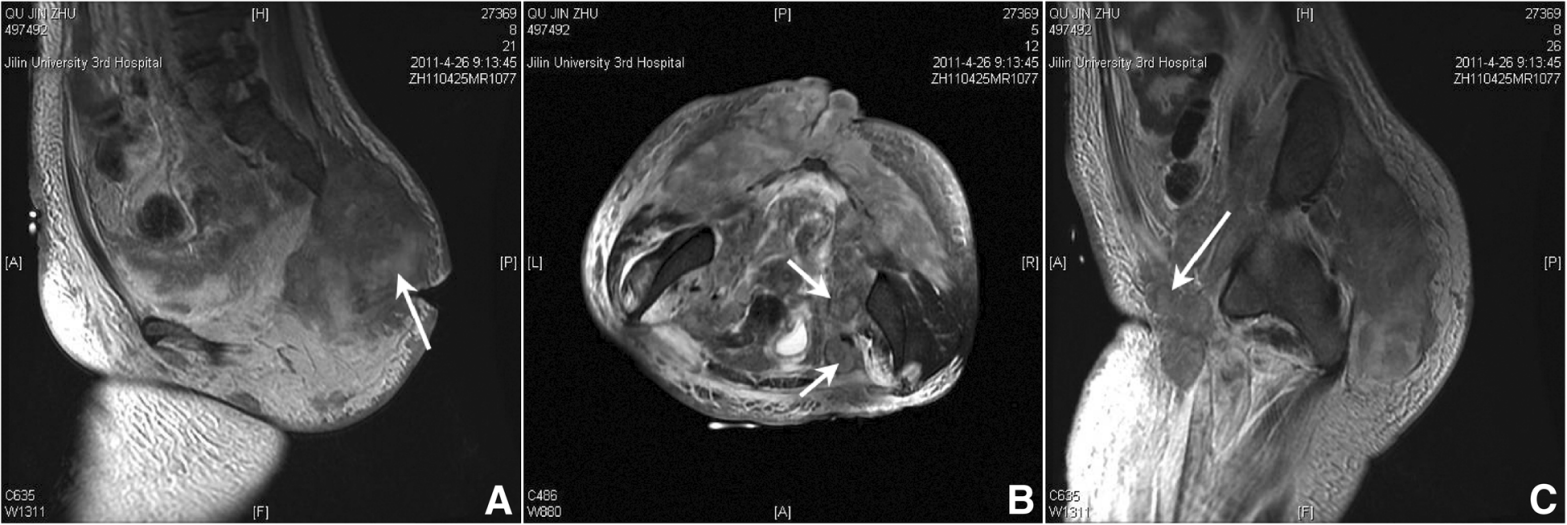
Magnetic resonance images revealing a large heterogeneous, irregular mass in the buttocks (A, arrow) and involvement of the pelvic (B, arrows) and inguinal lymph nodes (C, arrow).
